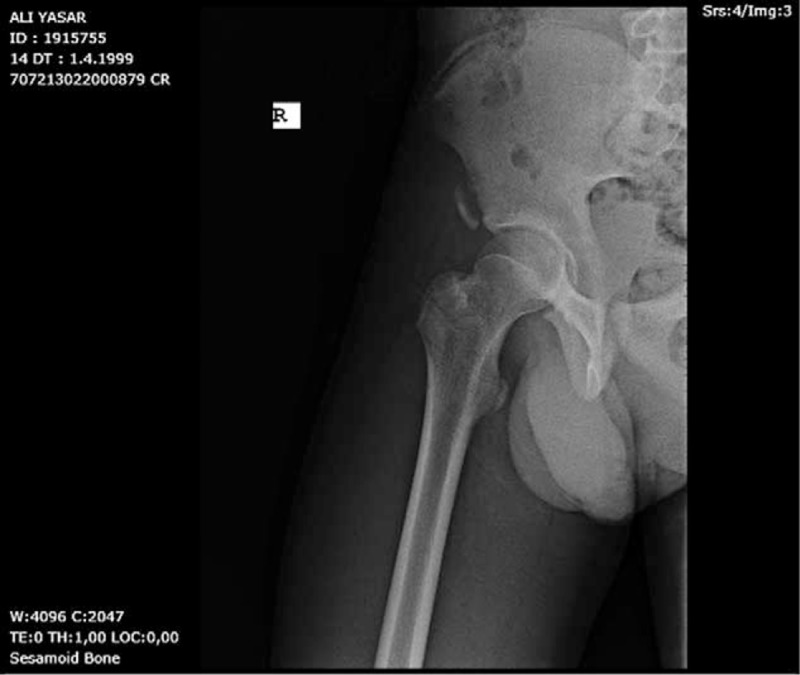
Pelvis anteroposterior x-ray shows a right anterior inferior iliac spine avulsion fracture in case 1.
